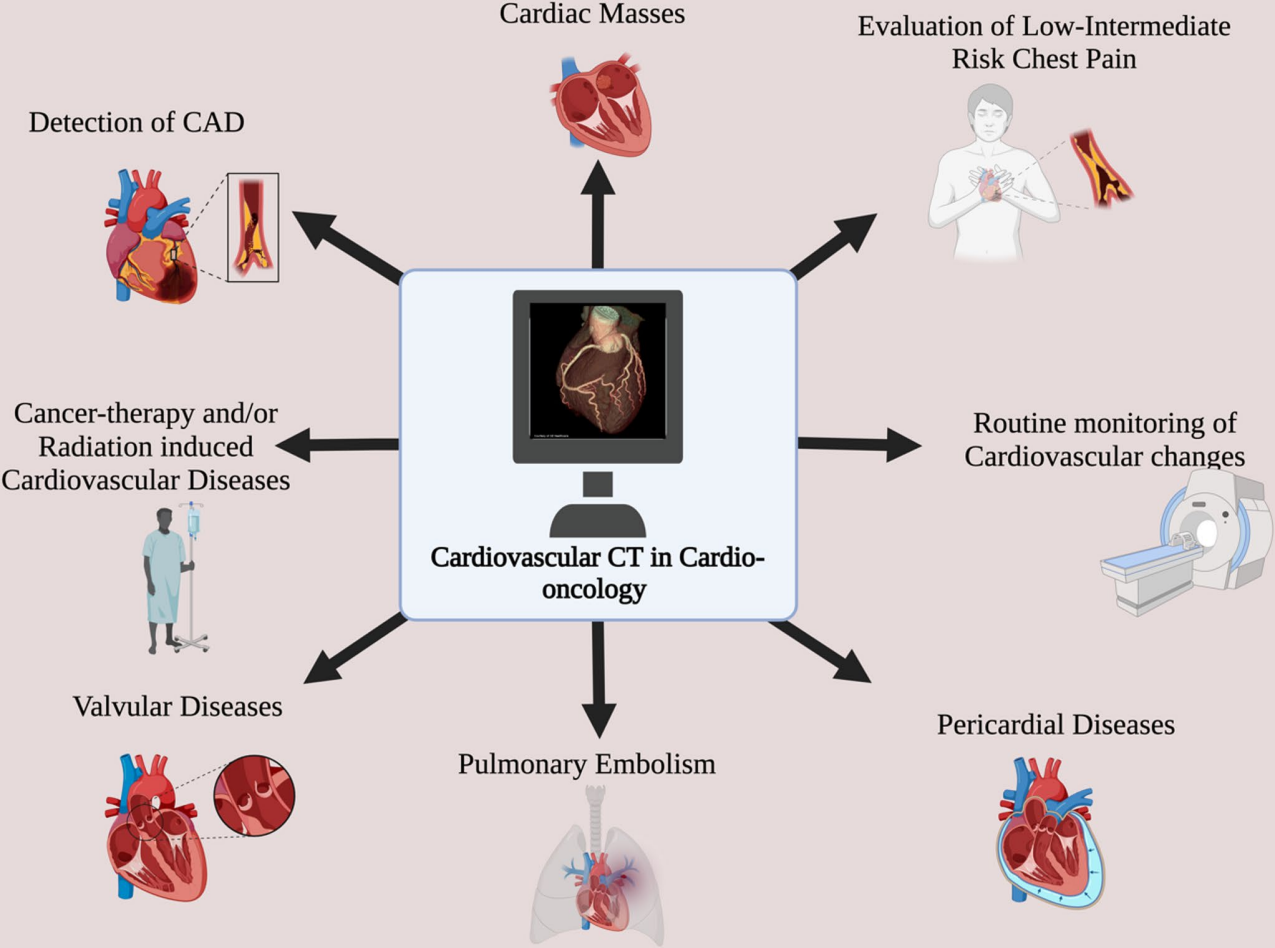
Cardiovascular CT Applications in Cardio-oncology. Created in BioRender. Erbay, M. (2023) BioRender.com/d50a424
